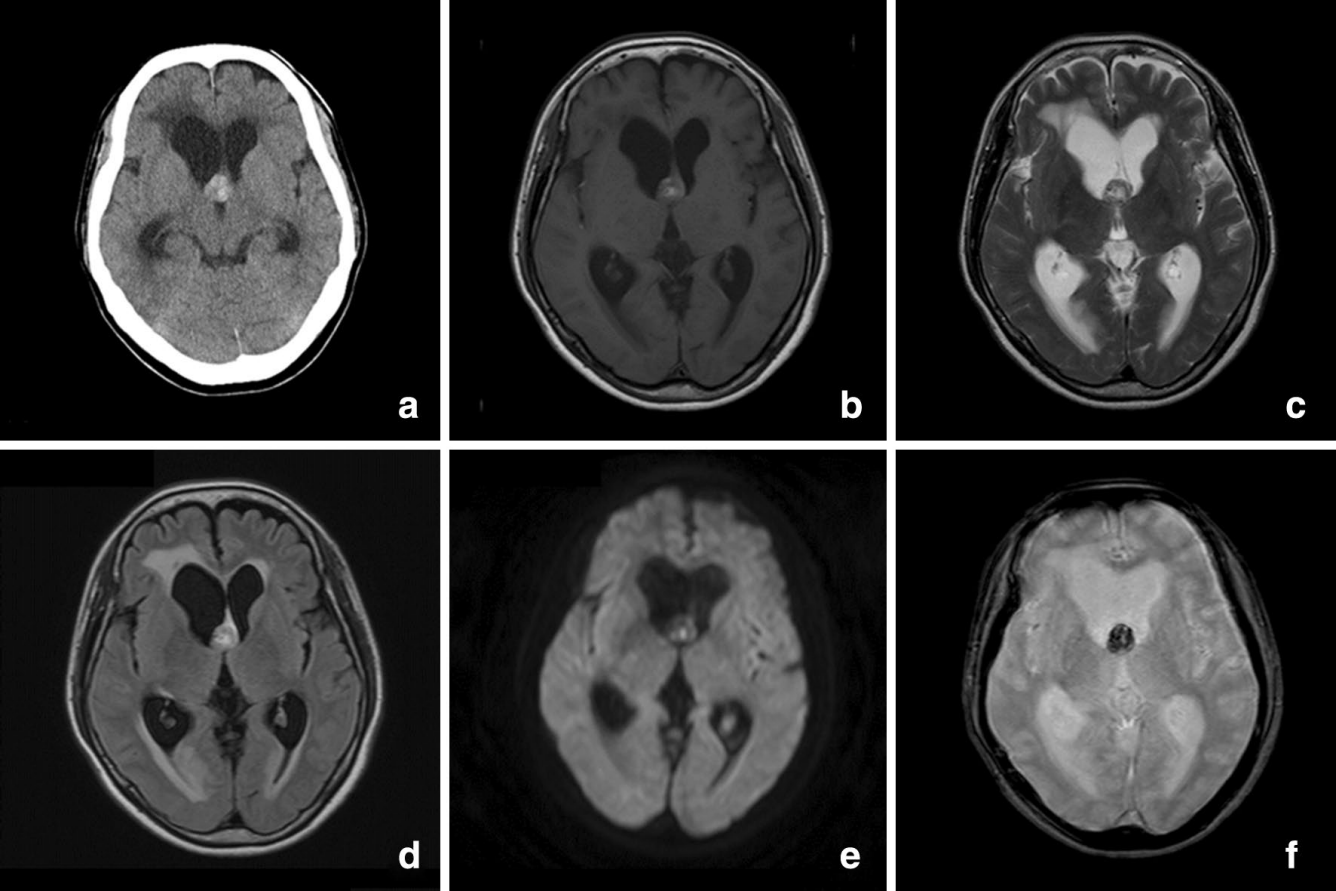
Computed tomography and magnetic resonance imagings. a There was a mild hyperintense 16-mm-diameter mass without calcification at the foramen of Monro causing obstructive hydrocephalus. b, c T1- and T2-weighted images showed the well-delineated mixed-signal heterogeneous core. The typical peripheral hemosiderin rim of low signal intensity was not seen on T2-weighted imaging. d No perilesional edema was presented on the fluid-attenuated inversion recovery magnetic resonance image. e Diffusion-weighted imaging showed an isointense mass; only a portion of the mass was hyperintense. f T2-star-weighted imaging showed a hypointense mass
